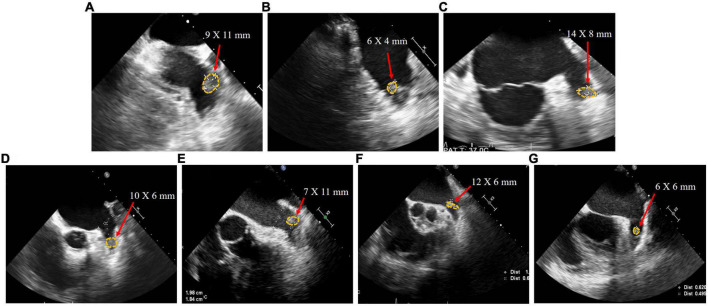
Transesophageal echocardiography images of patient (A–G) with left atrial appendage thrombus before left atrial appendage closure.
